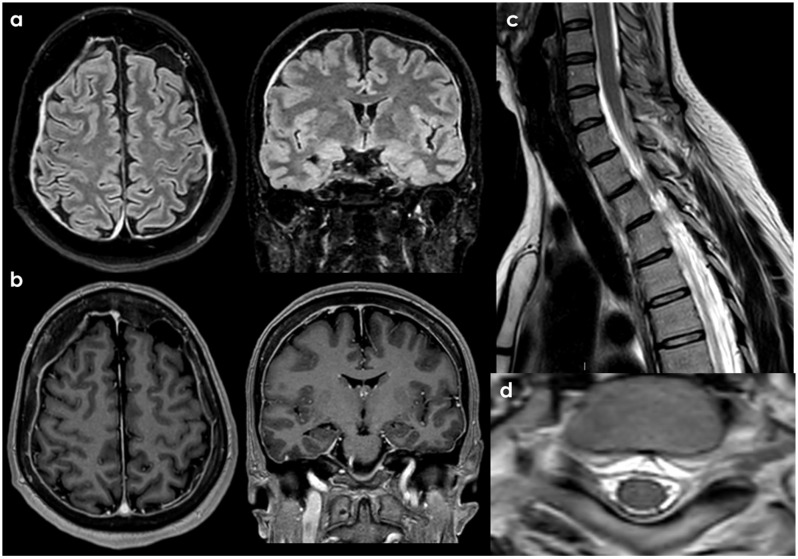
Brain and spine MRI findings in a patient with SIH (a,b and c,d, respectively). In (a), axial and coronal T2 Fluid Attenuated Inversion Recovery (FLAIR) images are proposed with a spontaneous pachymeningeal hyperintensity with contrast enhancement in the corresponding post-contrast T1W images (b). In the sagittal and axial T2W spine MRI (c and d, respectively), a VLISFC is evident. SIH: spontaneous intracranial hypotension; VLISFC: ventral longitudinal intraspinal fluid collection.
